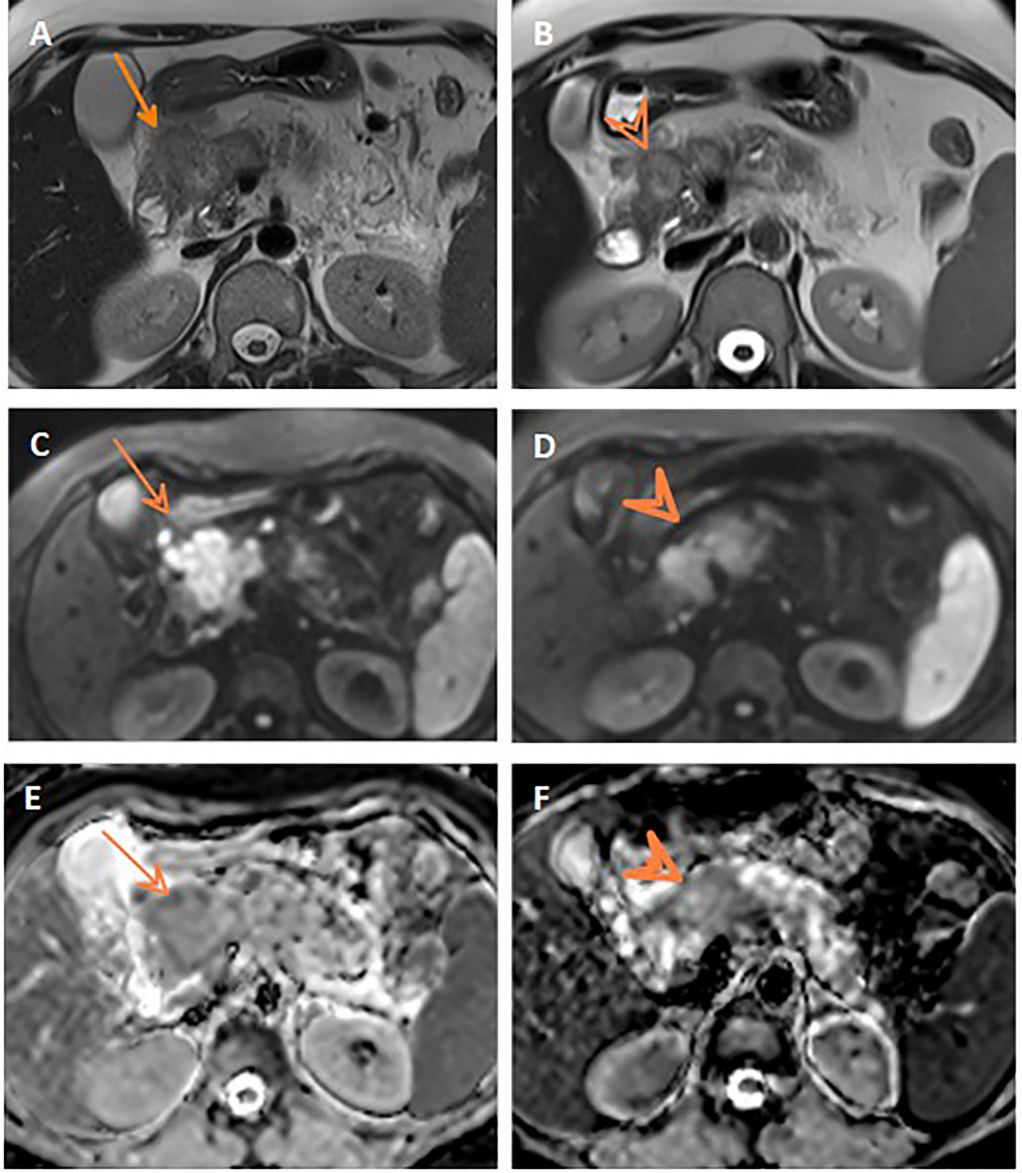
Discordant response between ADC and RECIST. Axial T2WI (A) shows progression of the pancreatic mass after neoadjuvant therapy compared to initial axial T2WI (B). Post-treatment and initial DWIs (C, D, respectively) show corresponding restricted DWI of the mass. Post-treatment and initial ADC maps (E, F, respectively) show comparable ADC values of the mass on both studies.
